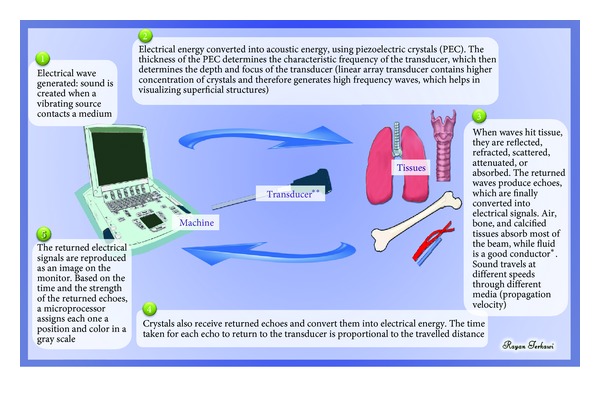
Concept of ultrasonography: *tissues that allow the beam to pass easily (e.g., containing fluids or blood) create only little echo (hypoechoic) and appear black on the screen, while tissues that allows less beam to pass (e.g., fat and bone) create stronger echoes (hyperechoic) and thus appear white on the screen; linear transducers have a higher frequency (10–15 MHz) and are usually used for superficial structures; curved transducers have a lower frequency (4–8 MHz) and are usually used for deeper structures.
